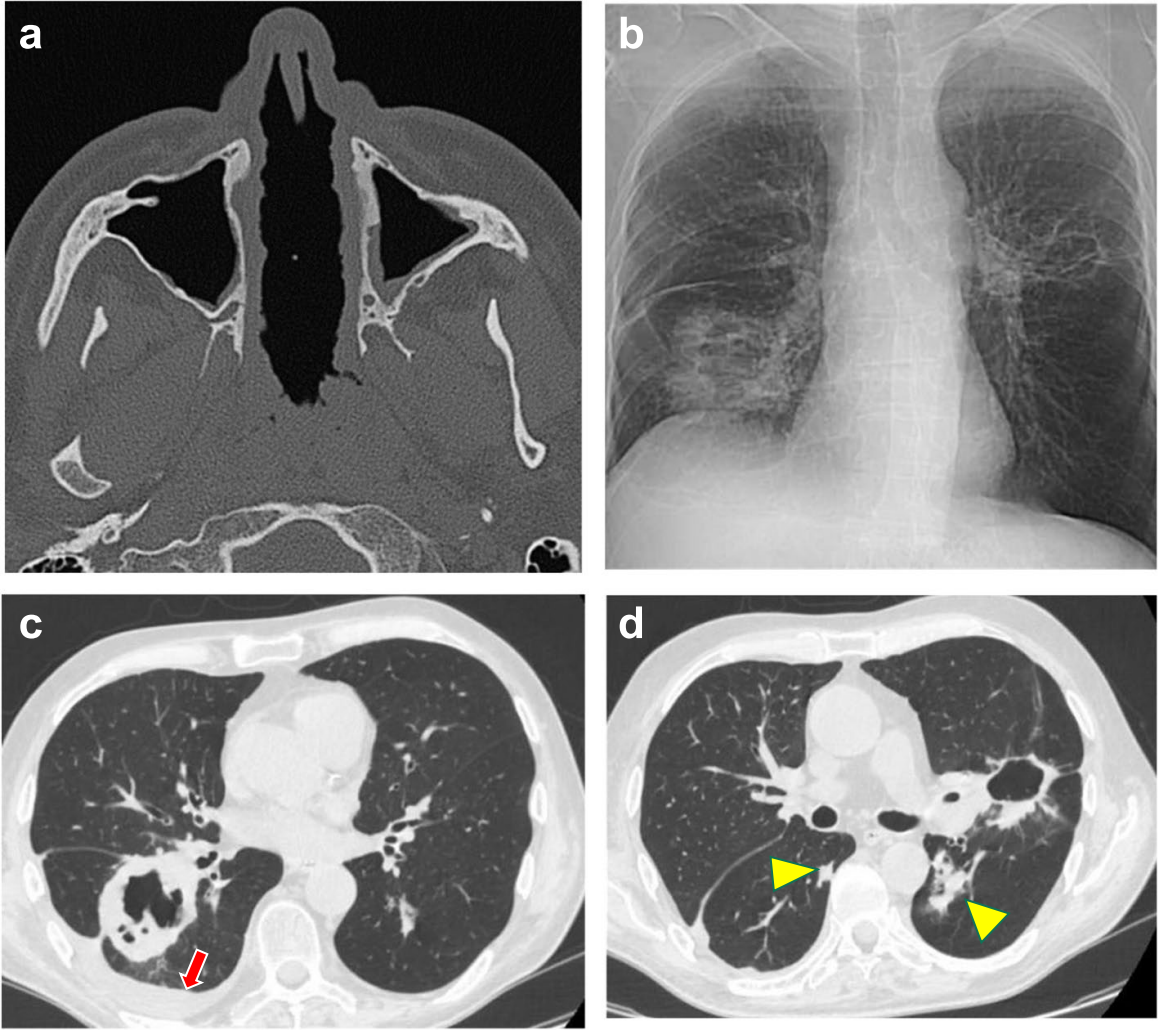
a) Necrotizing perforation of the nasal septum (patient 16 of Table 1). Axial CT demonstrates the nearly complete absence of the nasal septum. The mucosa appears thickened and nodular. b) Chest X-ray (patient 11 of Table 1), showing a large cavitary nodule with uneven and thickened walls in the right basal area and interlobar imbibition. An additional peri-hilar cavitary lesion with a clean, thin wall is also recognizable in the left lung. c) Chest CT confirms the presence of a cavitary granulomatous lesion with an anfractuous inner wall close to the right hilum and pulmonary fissure. The red arrow points to a modest layer of pleural effusion. d) The cavitary lesion close to the left hilum has a thinner, almost regular wall. Additional nodules of variable size are present in both lungs (yellow arrowheads)
